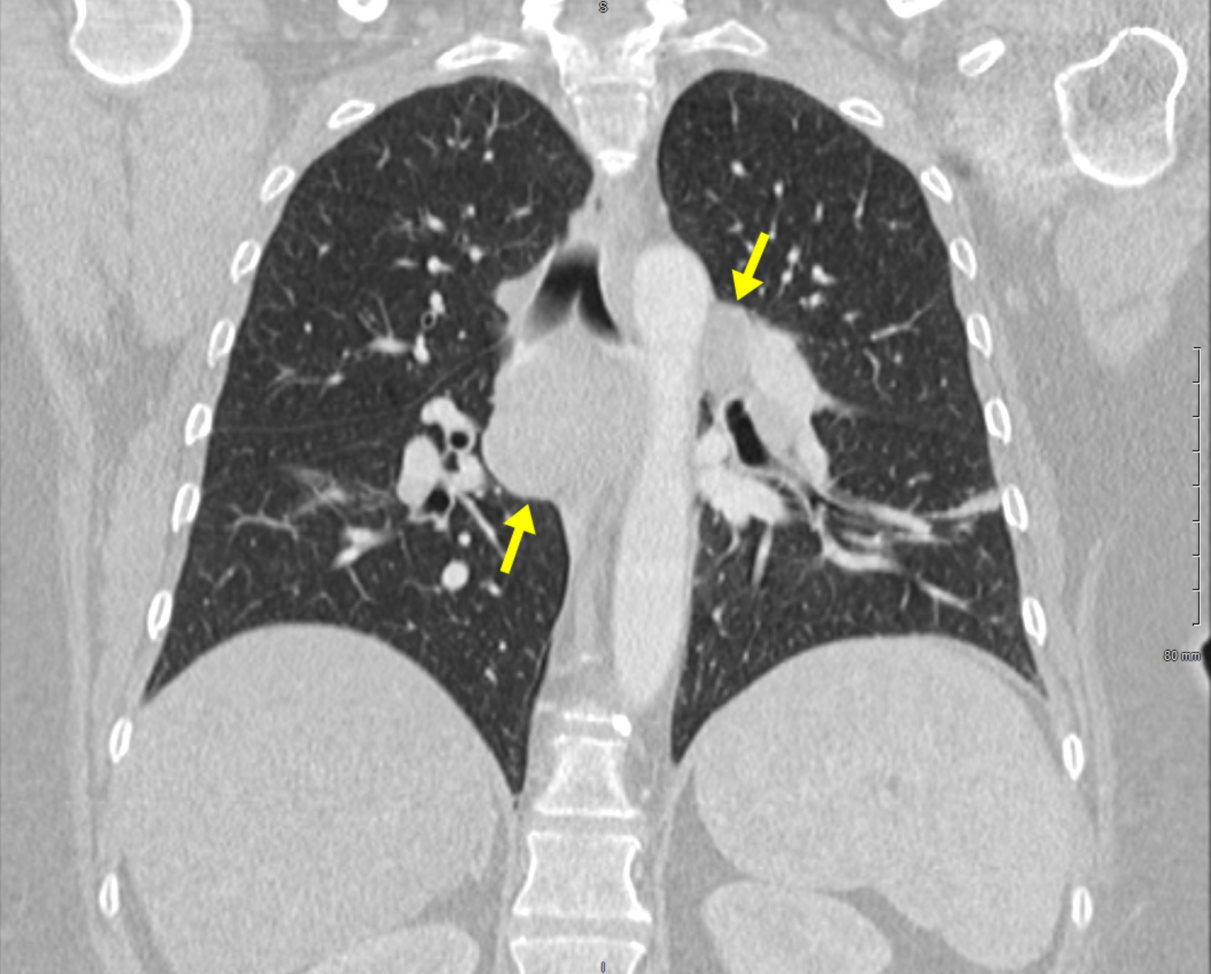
Computed tomography (CT) of the chest Numerous enlarged lymph nodes can be seen within the mediastinum. The largest is in the sub-carinal area measuring 3.1 cm x 4.0 cm. The second largest is in the right peritracheal area measuring 2.1 cm x 2.6 cm. Enlarged lymph nodes are also seen within each hilum, left greater than right.
